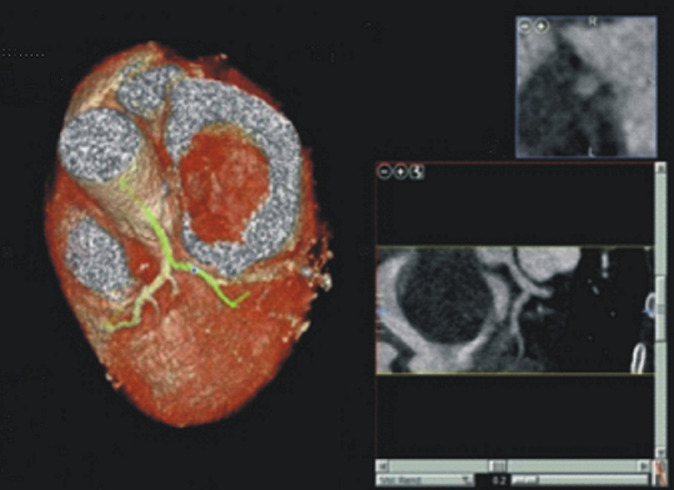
computed tomography coronary angiography, normal angiogram; a minimally enhancing hypodense lesion is seen in the lumen of the left atrium
